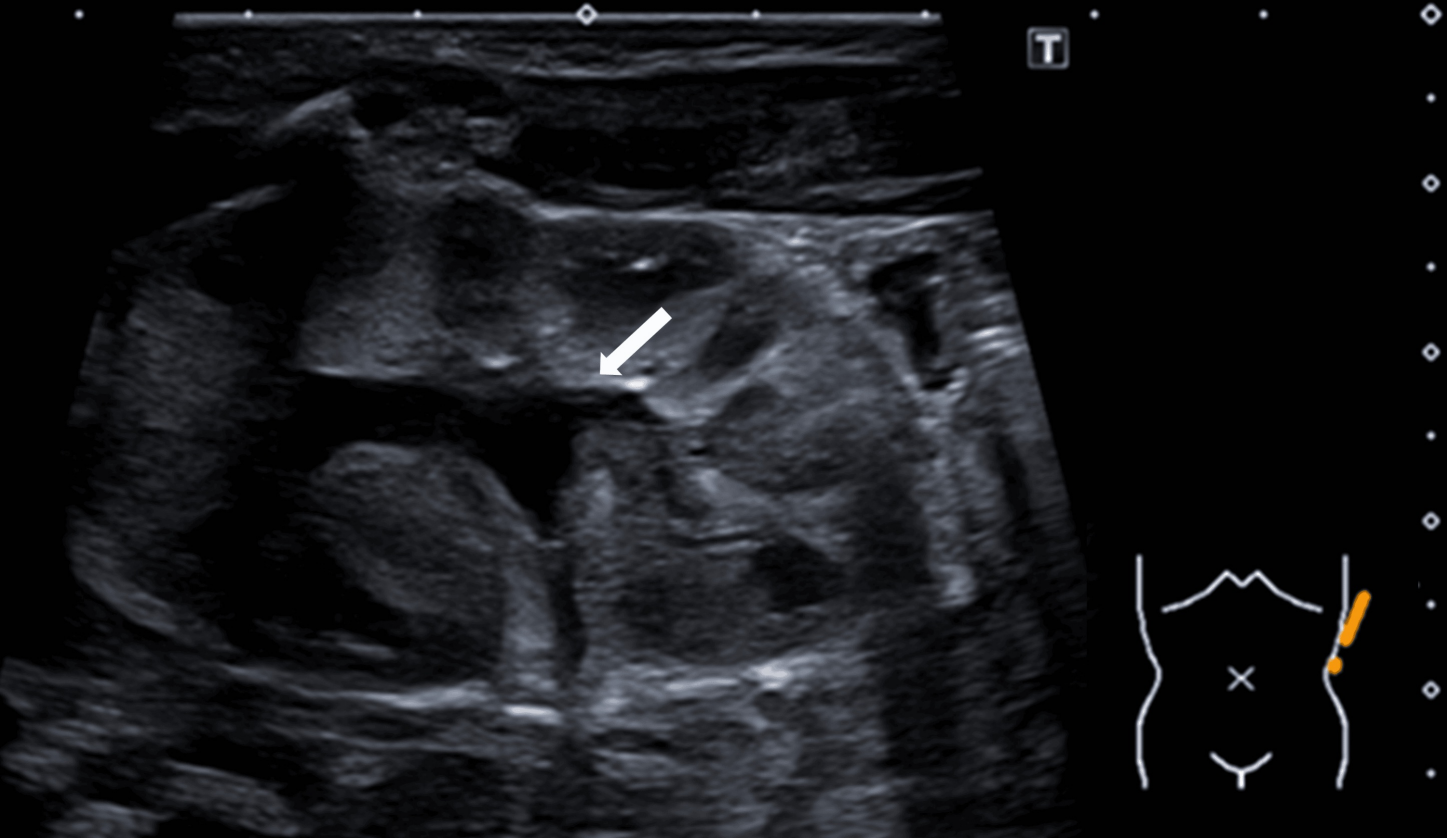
Abdominal ultrasound demonstrating grade 1 hydronephrosis of the left kidney
